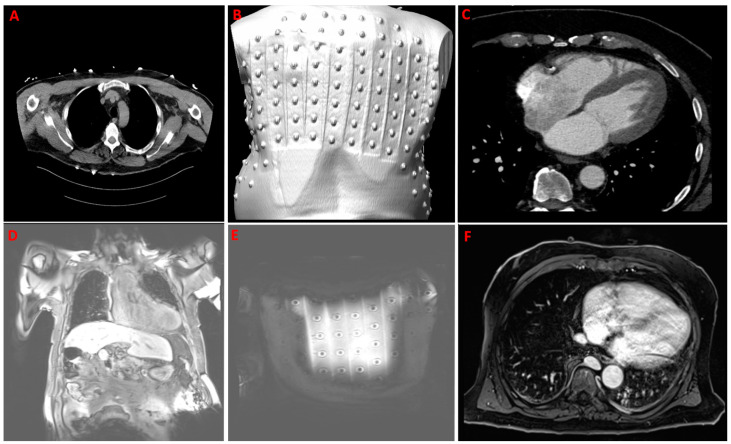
Upper row: example of recording computer tomography (CT). (A,B)—CT torso acquisition to capture applied metal 8-electrode strips (‘LungLowDose’ scan without contrast); (C)—contrast CT heart acquisition. Lower row: example of recording magnetic resonance imaging (MRI). (D,E)—torso series (t1_vibe_fs_cor_bh) in coronal plane to capture applied carbon MRI-compatible 8-electrode strips; (F)—MRI contrast heart acquisition with t1_vibe_fs_tra_p2_bh_iso_320 sequence.
